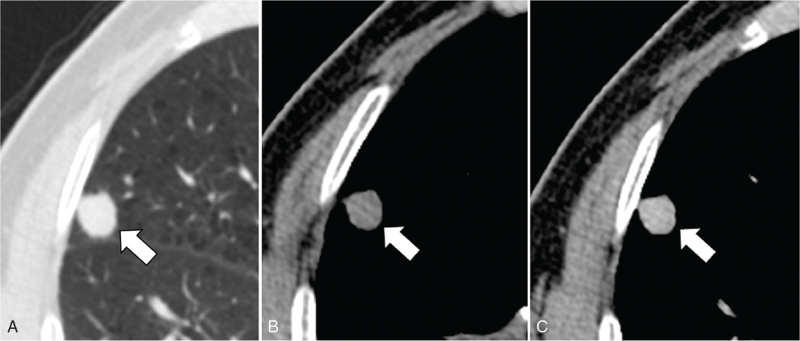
A 70-year-old man with basaloid squamous cell carcinoma of the lung. Unenhanced (A, B) and enhanced (C) CT scans show well-enhancing nodule with smooth contour (arrow) in right middle lobe periphery. CT = computed tomography.
